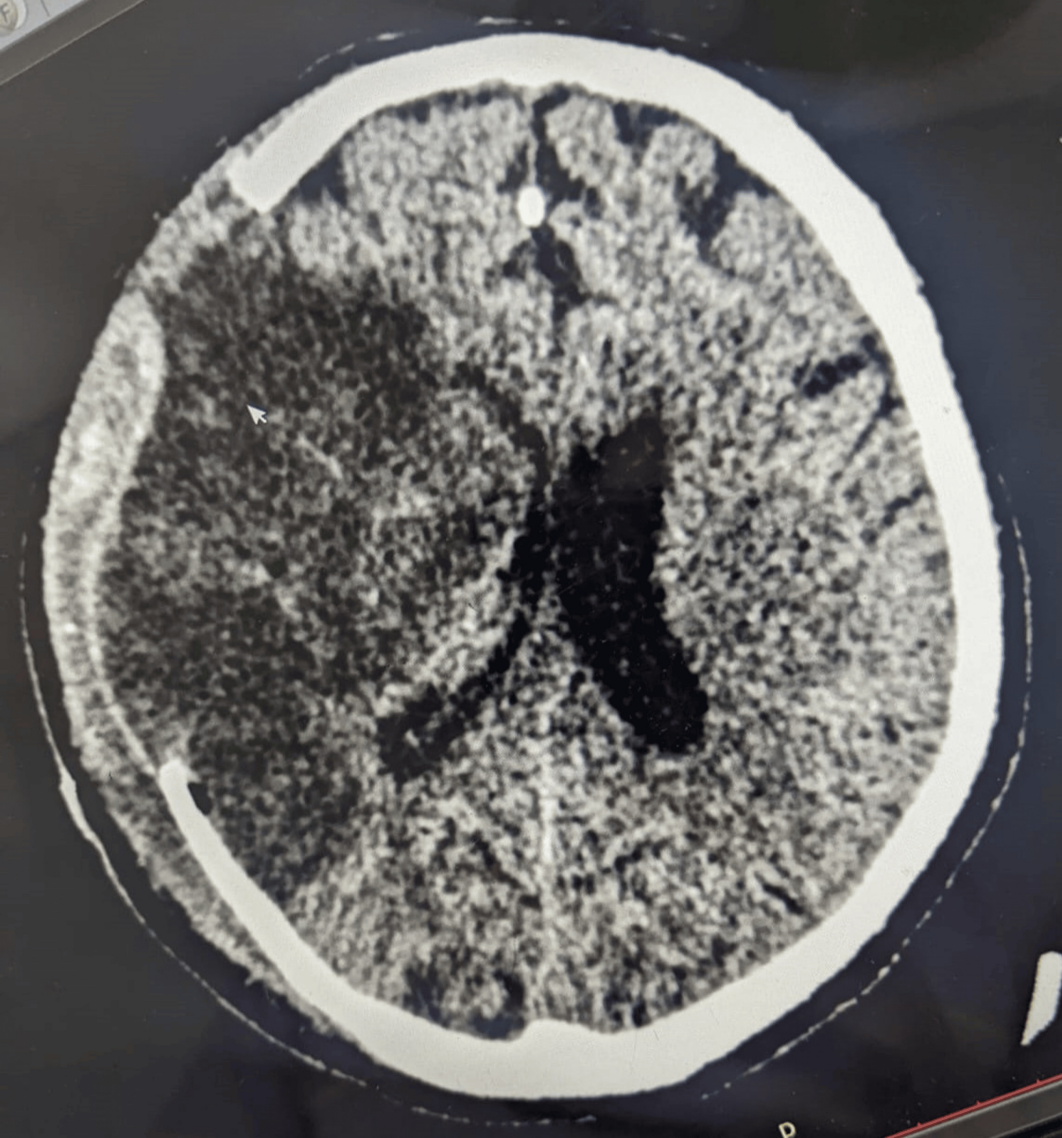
Non-contrast CT scan of the head revealed an infarct in the right middle cerebral artery territory.
